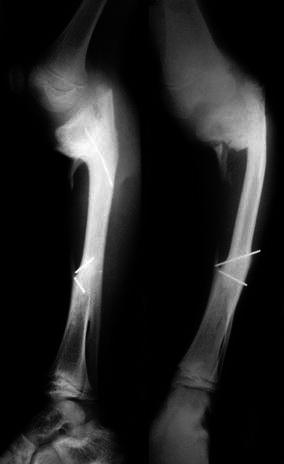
Lateral and antero-posterior radiographs of a 10-year-old boy showing marked varus and posterior angulation at the knee with proximal migration of the fibula, leading to shortening and stiffness of both knee and ankle joints
